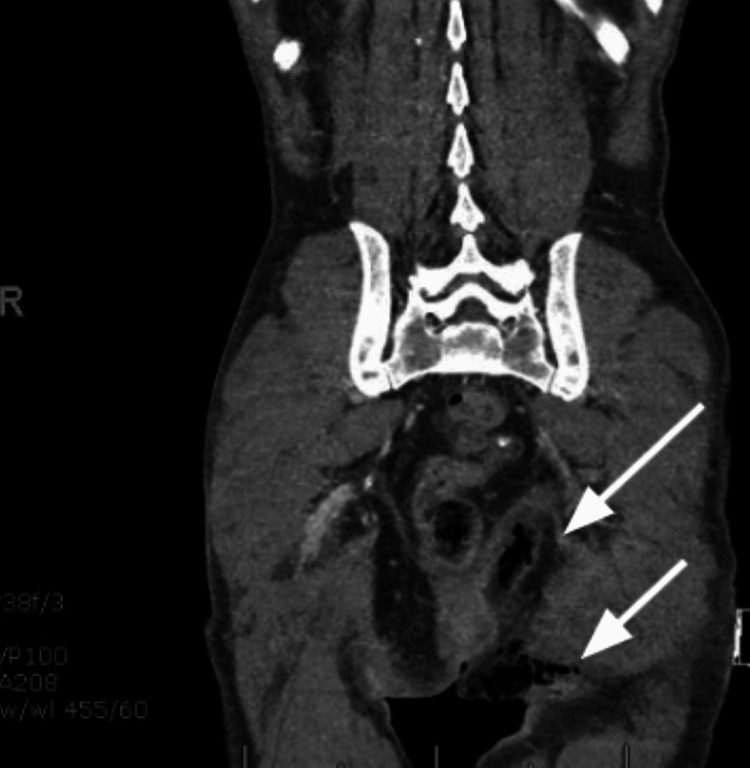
CT abdomen/pelvis shows soft tissue gas locules extending to the left buttock indicated by arrows.
